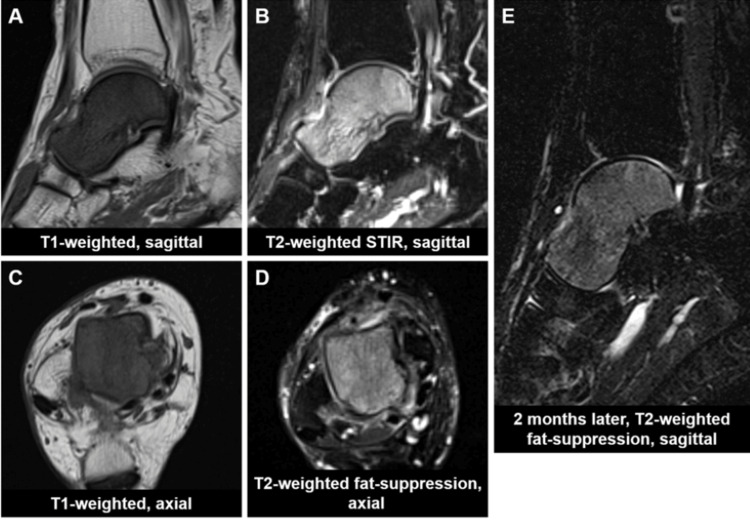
MRI at initial examination and two months after off-loading treatment STIR: short-tau inversion recovery
